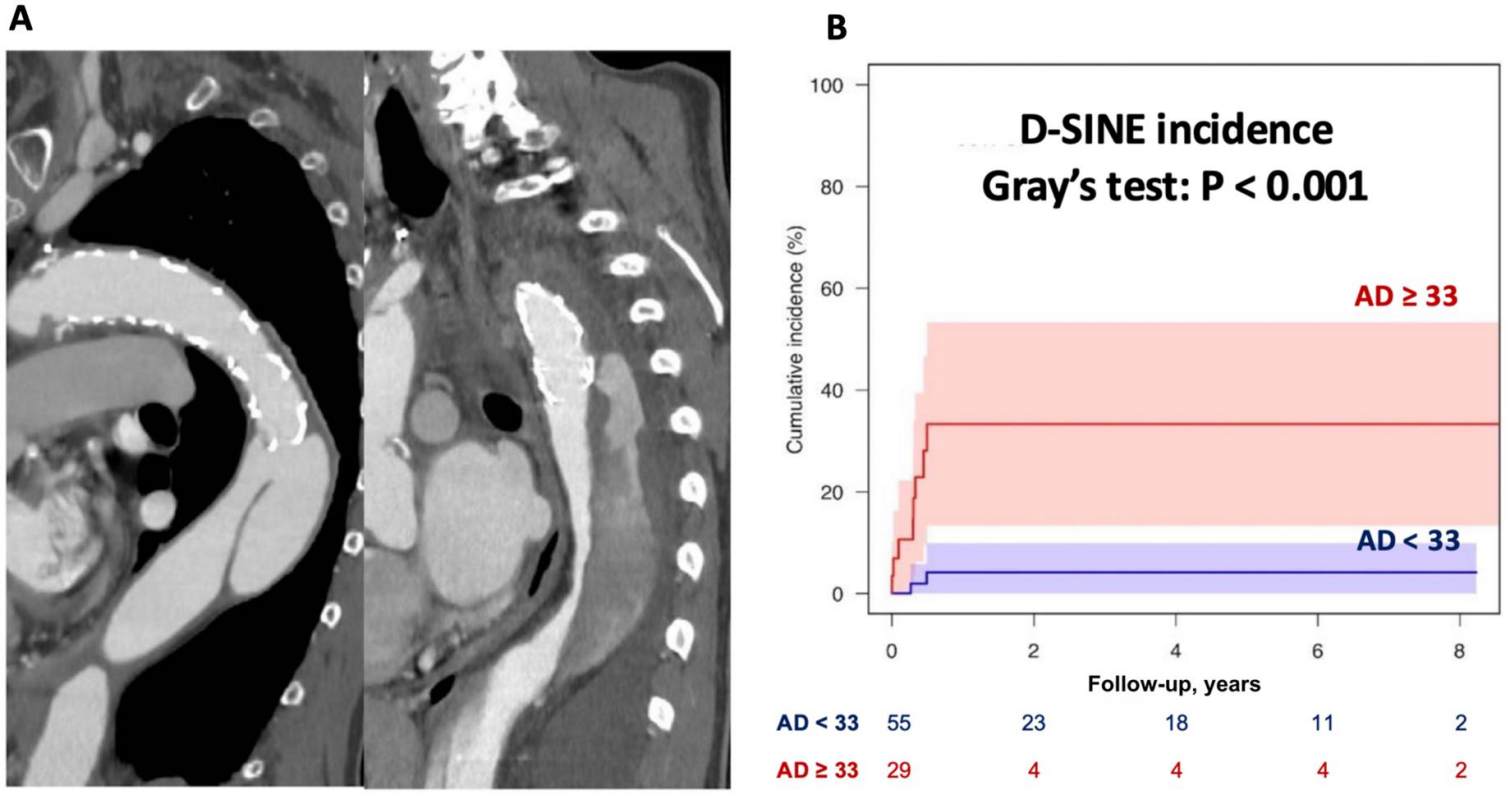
(A) Typical CT images of d-SINE following FET procedure. (B) Competing risk regression analysis for d-SINE occurrence, comparing descending aortic diameter <33 mm and ≥33 mm, with death as a competing event. d-SINE, distal stent graft-induced new entry; FET, frozen elephant trunk
